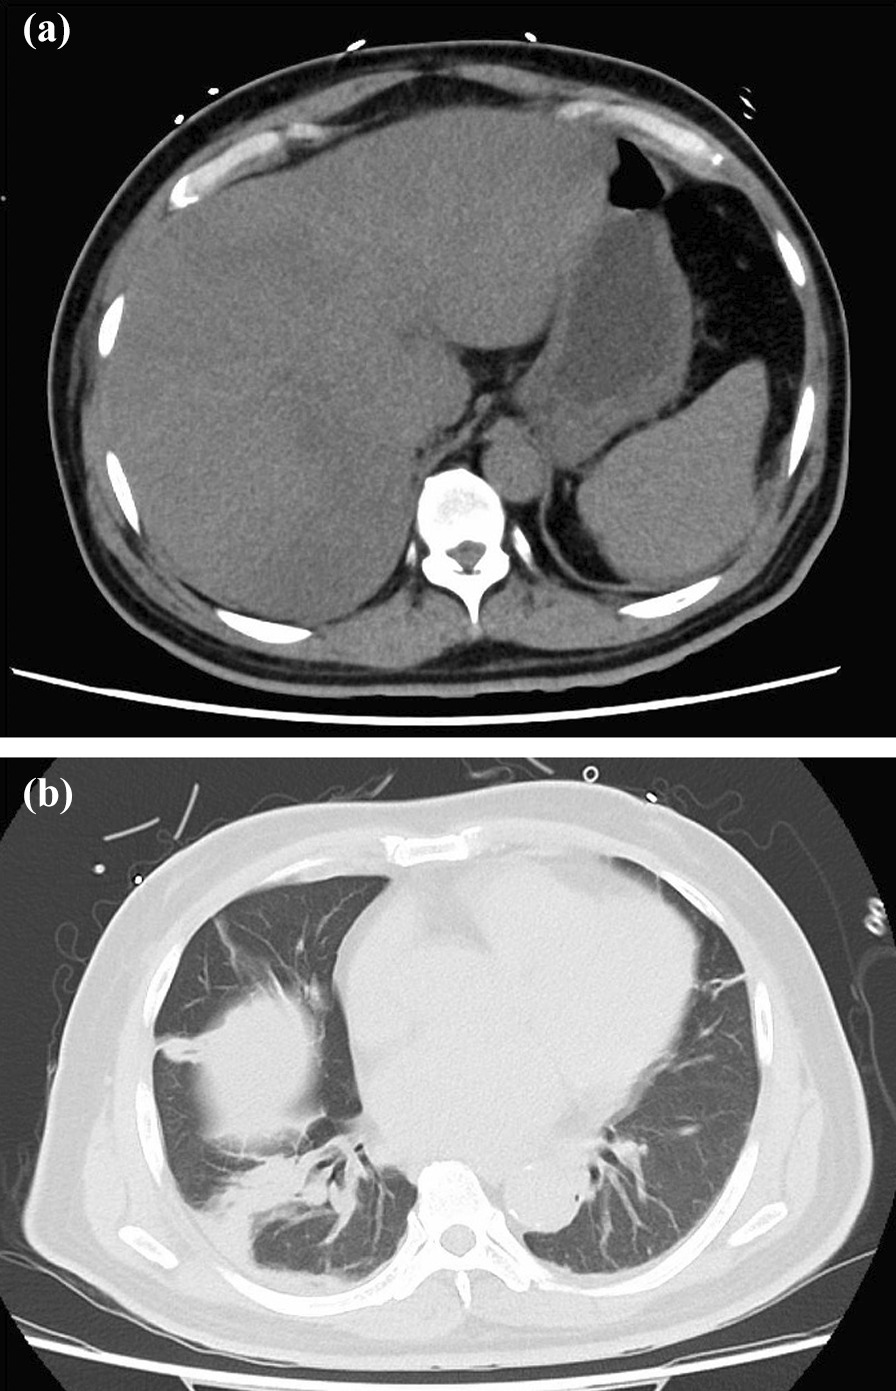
Plain chest and abdominal computed tomography scans were obtained on the day of transfer to our hospital. a Low absorbance of the liver, hepatomegaly, edematous thickening of the gallbladder, and splenomegaly are noted. b An infiltrative shadow on right lung is noted, which was diagnosed as pneumonia
